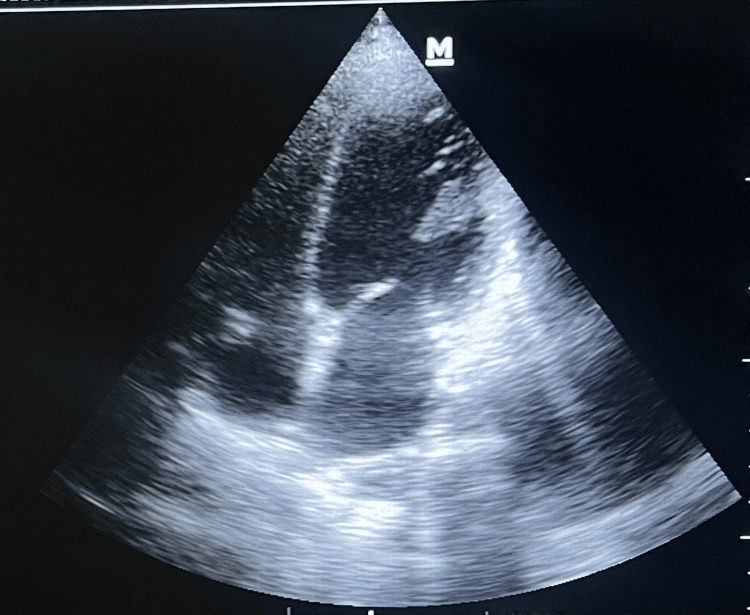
Follow-up 2D echo A repeat 2D echocardiogram shows marked improvement in left ventricular systolic function.
